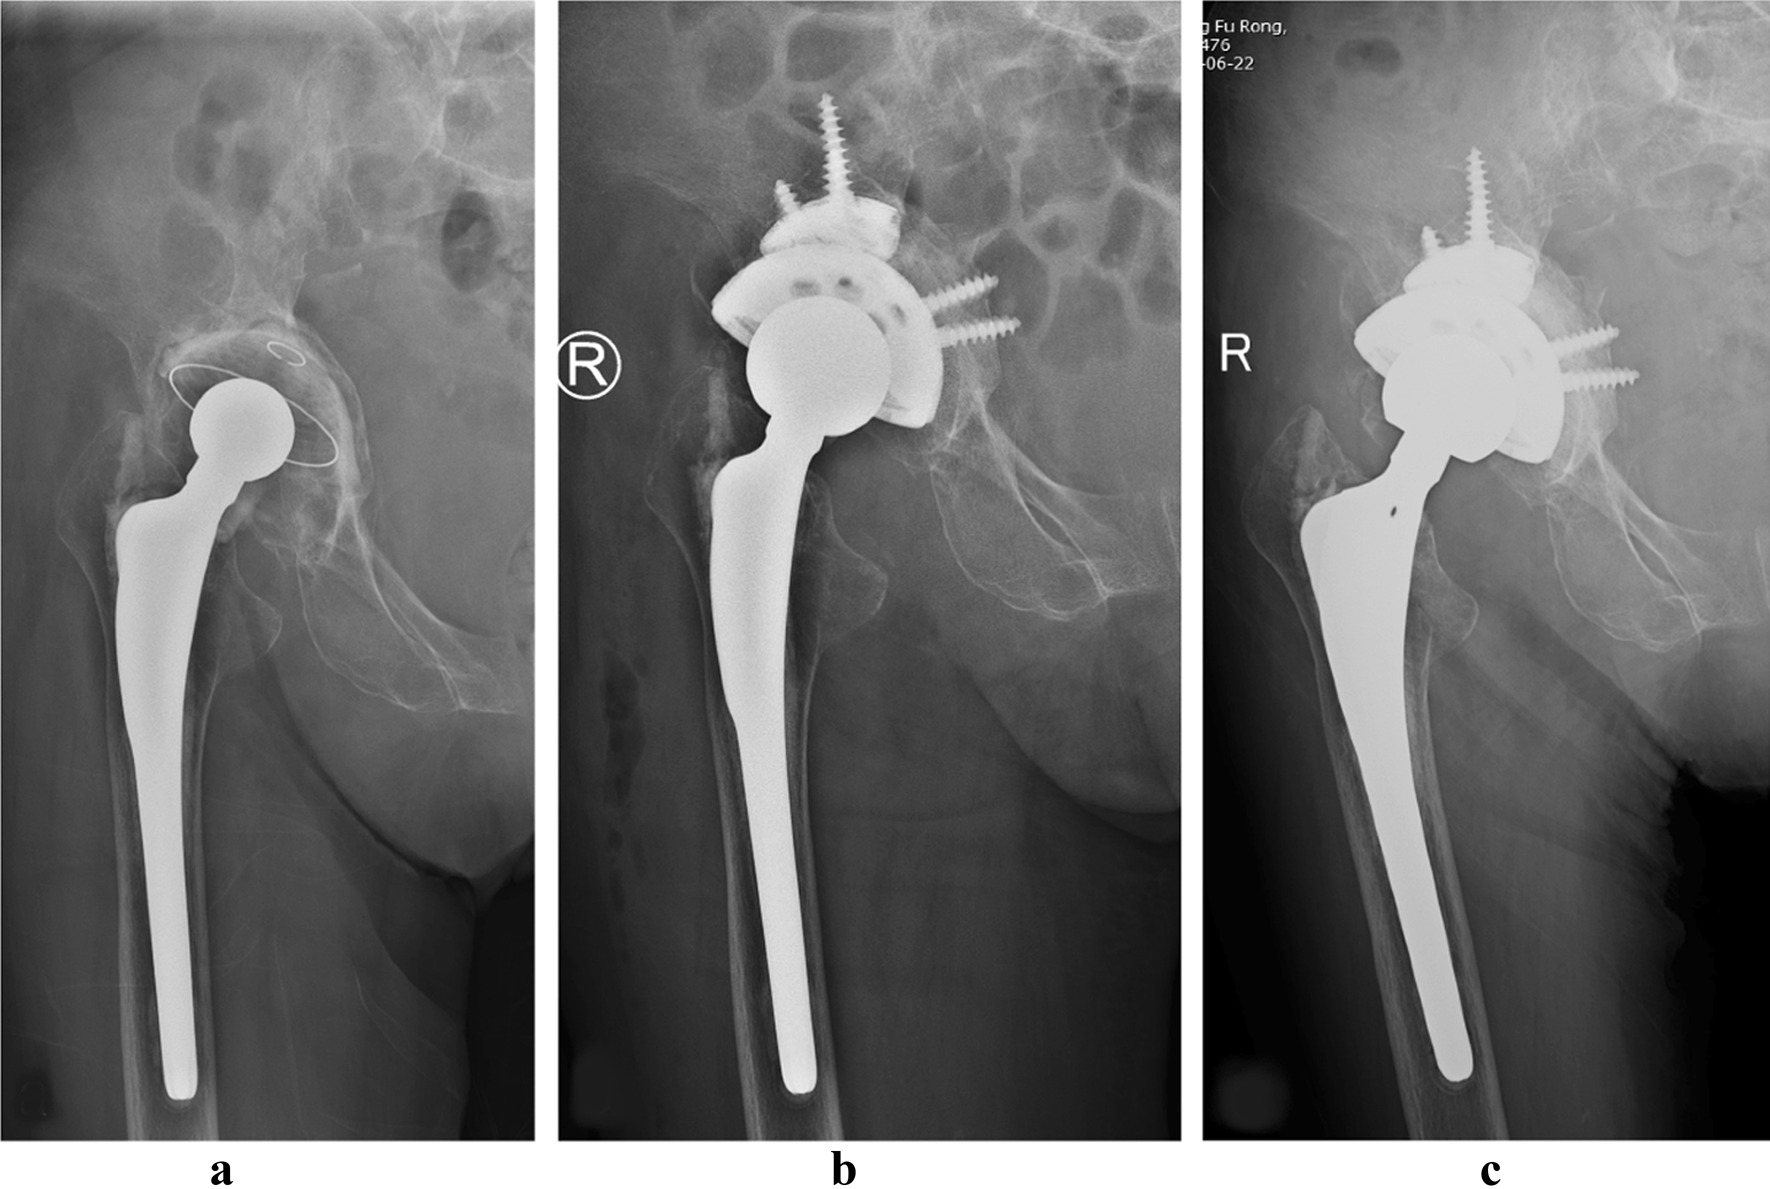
AP radiograph of an 82-year-old female patient with a type IIIB bone defect revised for aseptic loosening of the acetabular cup. a before surgery; b three days after revision surgery; c 72 months after revision surgery
